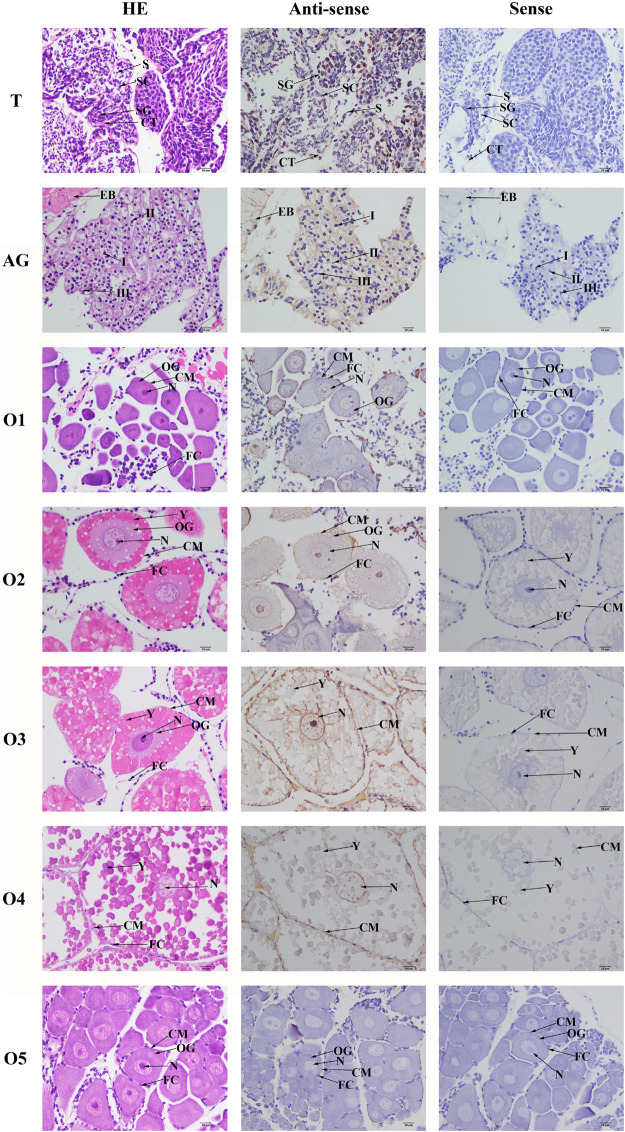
In situ* hybridization analysis of Mn-NF_k_Bα gene in the testis and androgenic gland from reproductive season, and different reproductive cycle of ovary of *M. nipponense*. SG, spermatogonia; SC, spermatocytes; S, sperms; CT, collected tissue; I, Stage I of androgenic gland cell; II, Stage II of androgenic gland cell; III, Stage III of androgenic gland cell; EB, ejaculatory bulb; OG, oogonium; OC, oocyte; CM, cytoplasmic membrane; N, nucleus; Y, yolk granule; and FC, follicle membrane. Scale bars = 20 μm.
